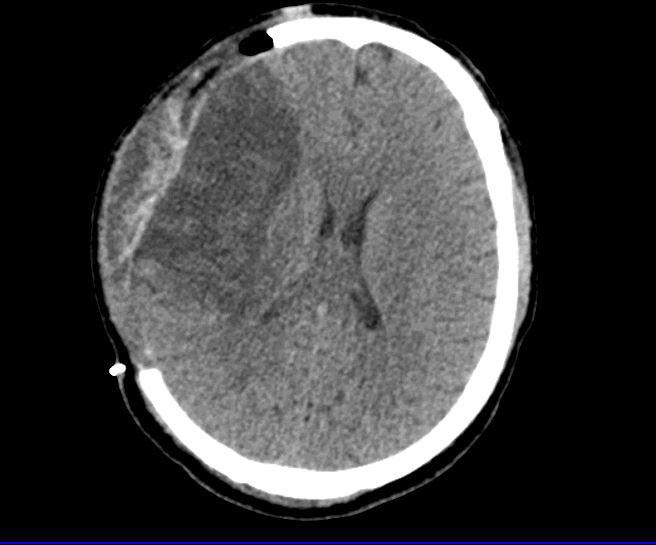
Post decompressive craniectomy CT head showing ischemic stroke in the right MCA territory with features of raised ICP.
